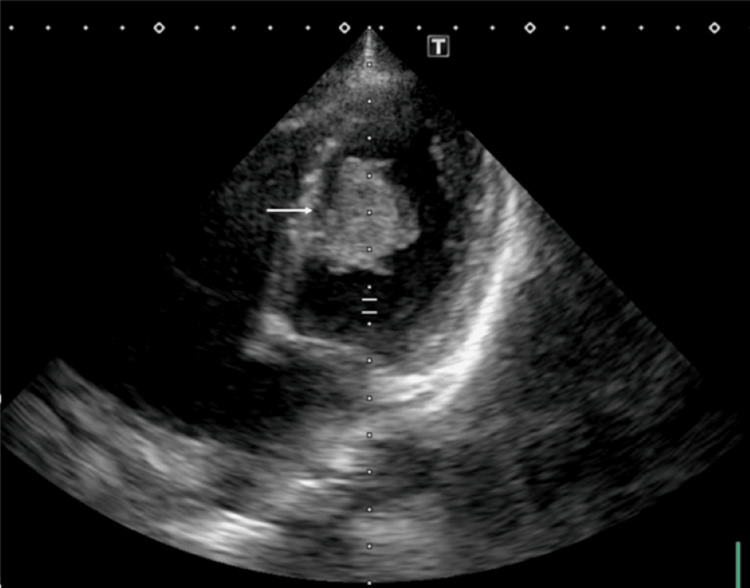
Pre-operative transthoracic echocardiography Approximately 30-mm, pedunculated mass with mobility in the left ventricle (arrow).
